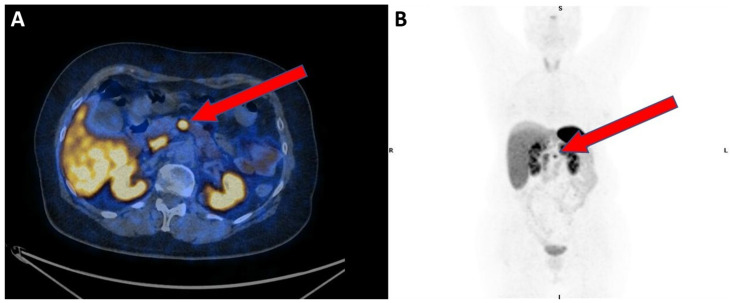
Presurgical [^68^Ga]Ga-DOTANOC PET/CT transaxial (A) and MIP (B) show focal and intense uptake in the primary pancreatic lesion (red arrows).
